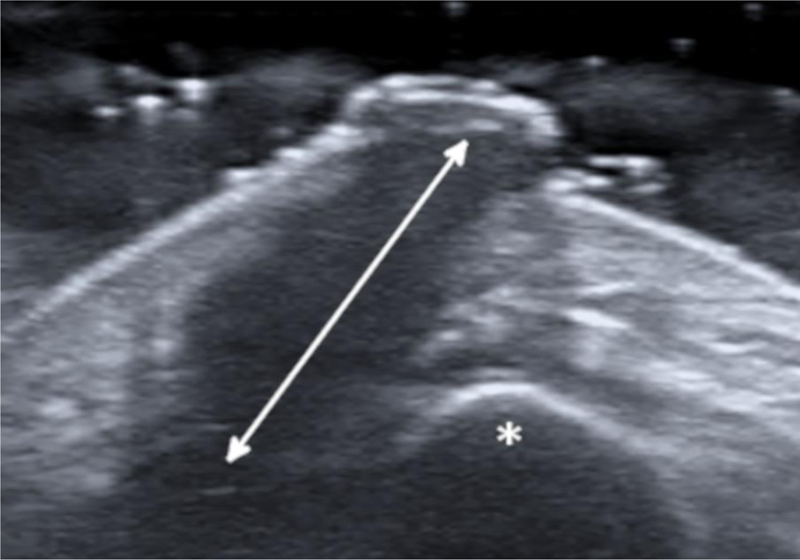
Ultrasonography examination reveals a wide hypoechoic band (arrow) extending through the subcutaneous tissue to the mandibular bone (asterisk).
